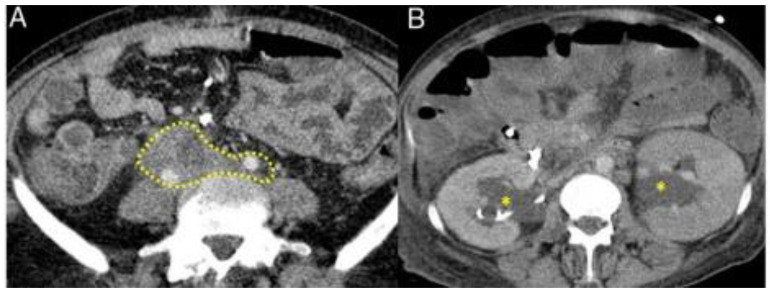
Typical features of retroperitoneal fibrosis in IgG4-RD seen on CT and MRI. Fibrotic process involving the ureters and iliac arteries in the abdomen (dashed mark, A) with hydronephrosis (asterisks, B). [Adapted] [23].
